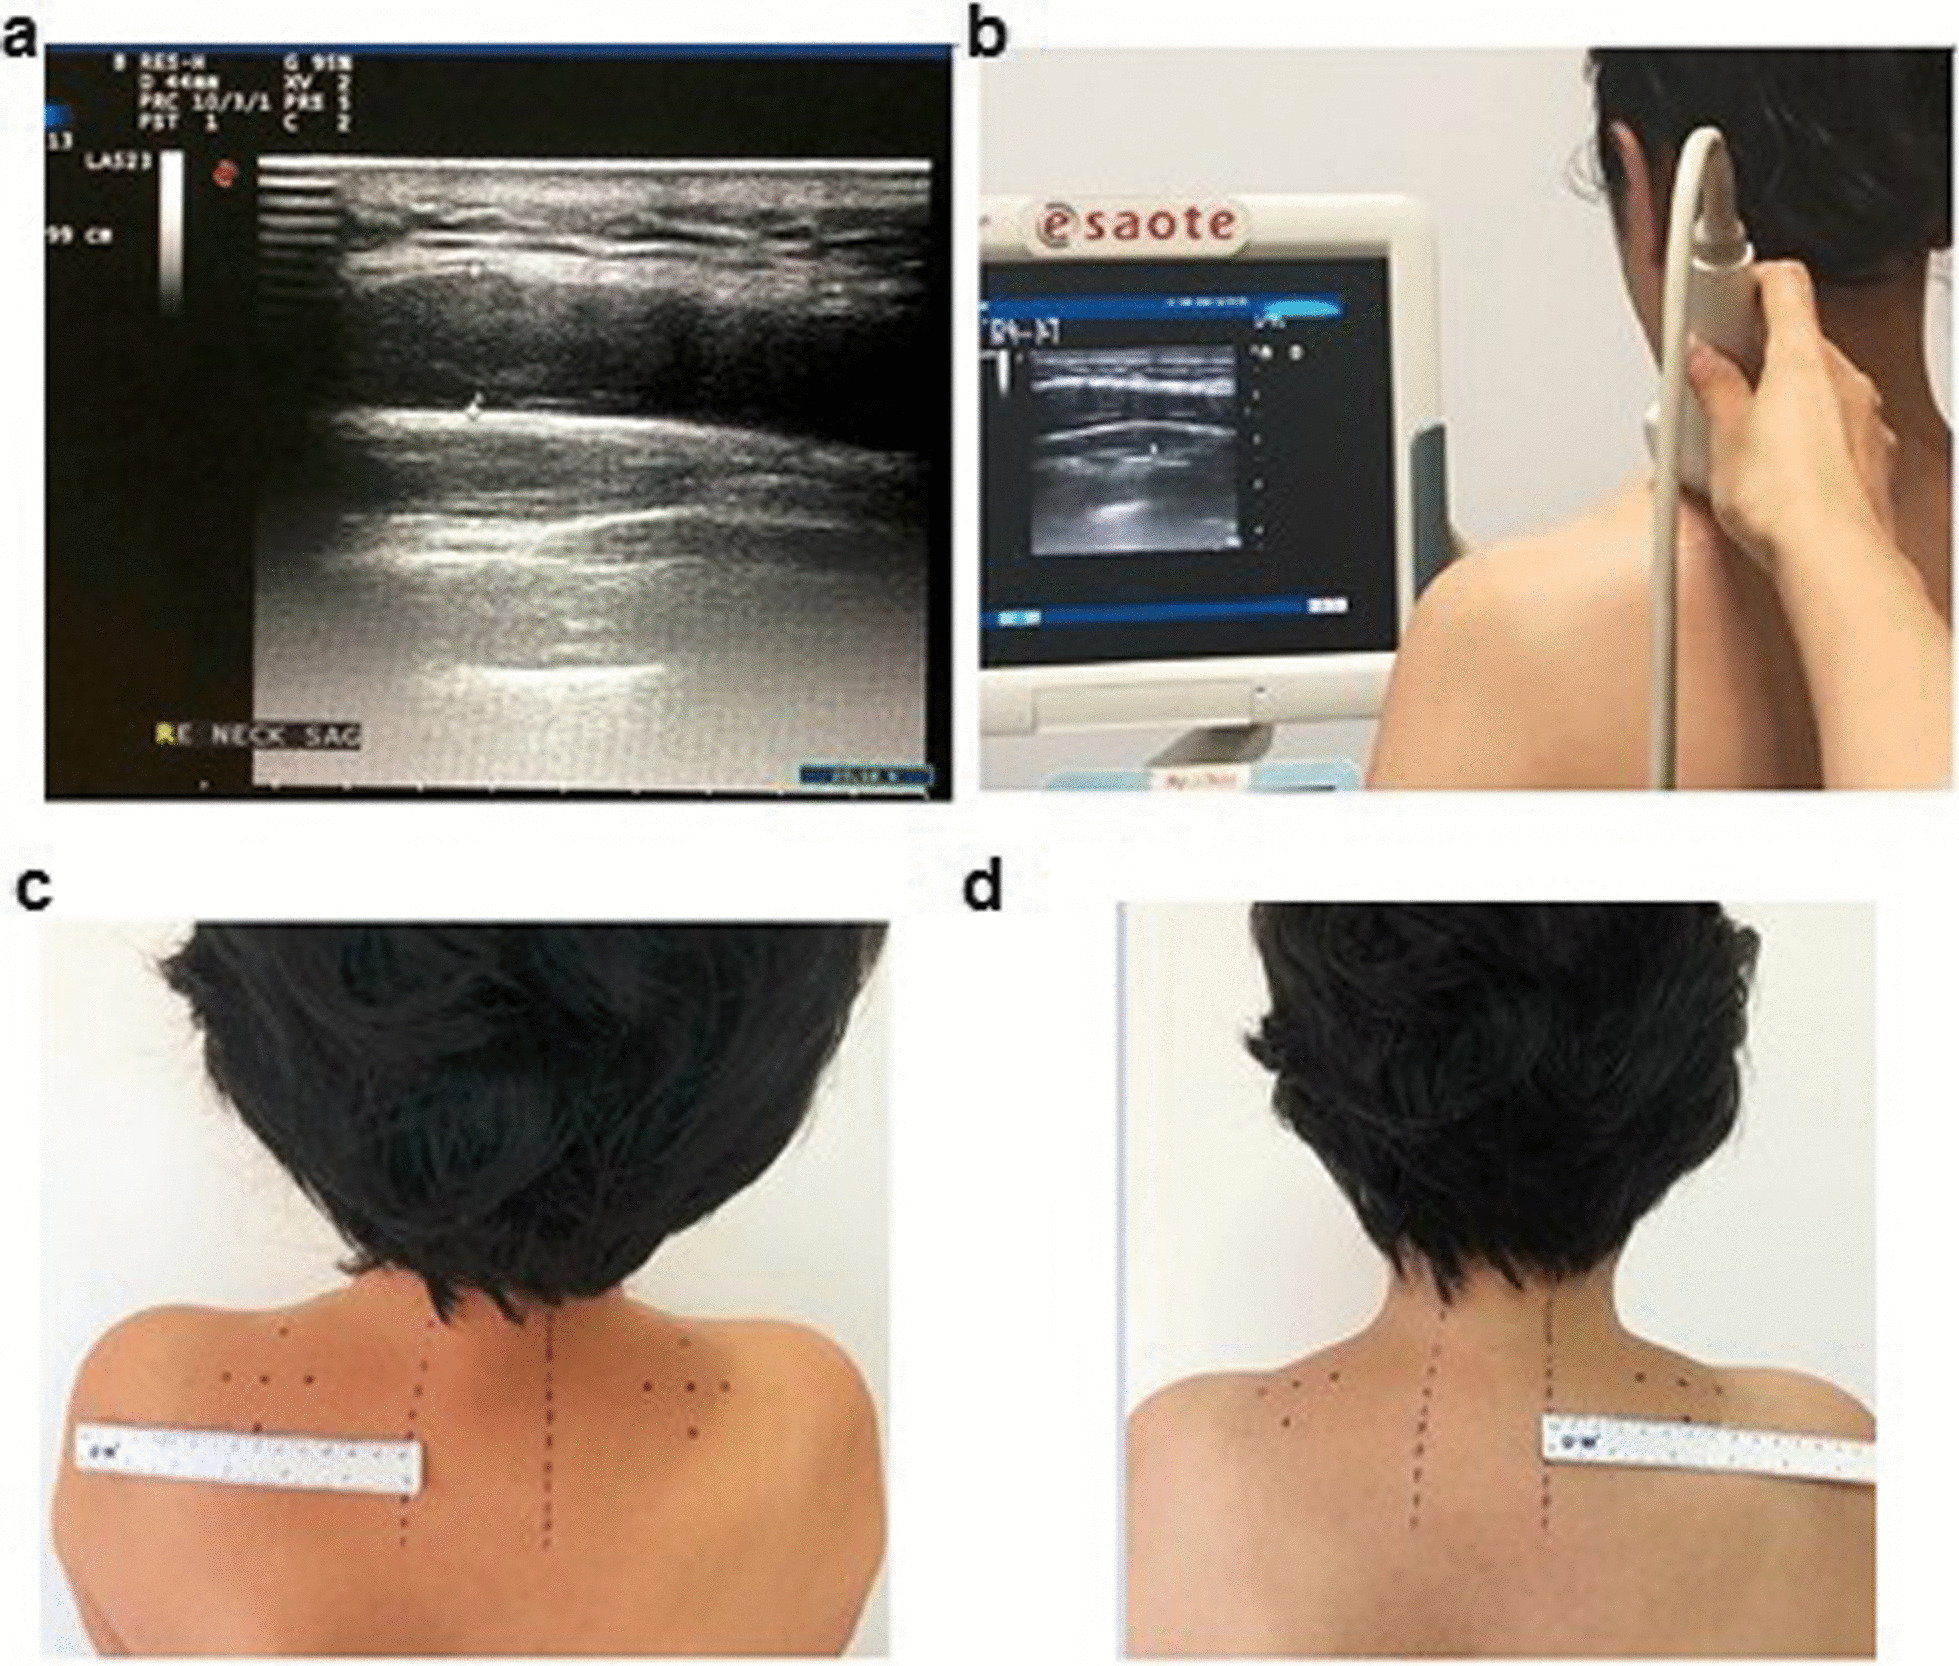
Measurement of muscle thickness and labeling of botulinum toxin type A (BOTOX) injected points. a, b The muscle thickness was measured by using the ultrasound scanner Esaote MyLab Twice; c the five points at left trapezius muscle for BOTOX administration; d the five points at right trapezius muscle for BOTOX administration
